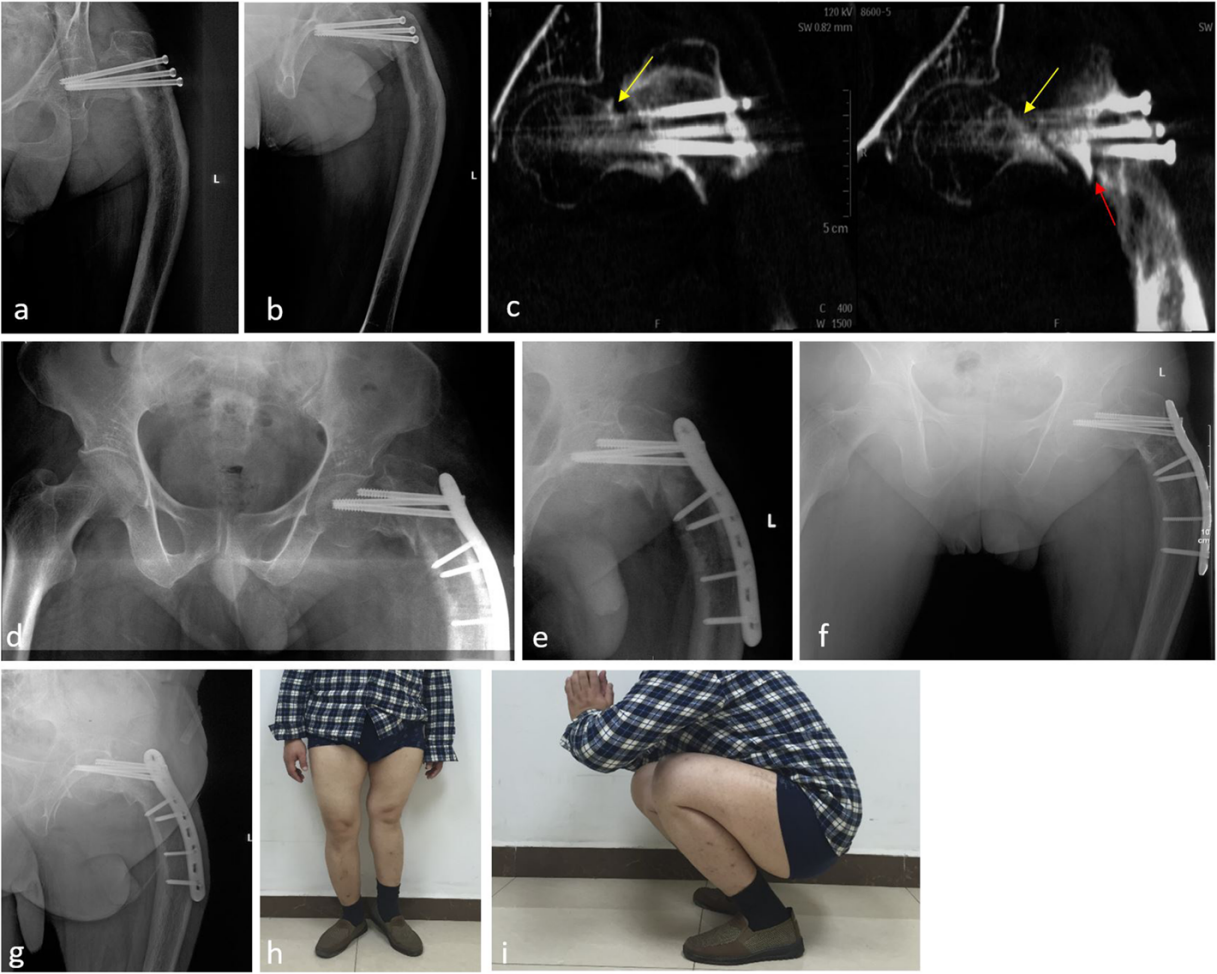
The patient was a 41-year-old blind male who accidently fell from a height, which caused left hip pain and limited activity. Two months prior, he had presented with ipsilateral femoral neck fracture due to falling from a standing height and was treated with closed reduction and cannulated screws fixation. Additionally, 34 years ago, the left thigh was subjected to a proximal femoral fracture, which was conservatively treated. A preoperative anteroposterior X-ray (a) and a lateral X-ray (b) demonstrated a left femoral intertrochanteric fracture, cannulated screws fixation for previous femoral neck fracture, and a proximal femoral angulation deformity due to the injury 34 years ago. CT radiographs (c) indicated a clear fracture line of the left femoral neck fracture (yellow arrow) and the ipsilateral femoral intertrochanteric fracture (red arrow). The cannulated screws were removed and the reshaped proximal femoral locking compression plate was used for fixation. Postoperative X-ray radiographs of the anteroposterior (d) and lateral view (e) 2 days after surgery. X-ray radiographs of the anteroposterior (f) and lateral view (g) 2 years after surgery indicated fracture union. The general functional outcomes at the 2-year follow-up (h, i)
